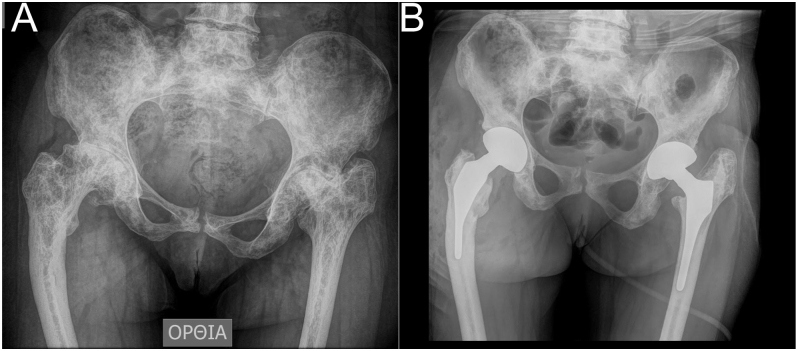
Preoperative (A) and immediate postoperative (B) radiographs of bilateral Hartofilakidis A dysplastic hips in a patient with sickle cell anaemia and abnormal proximal femoral anatomy, treated with hybrid total hip arthroplasty using custom-made stems at two separate stages.
